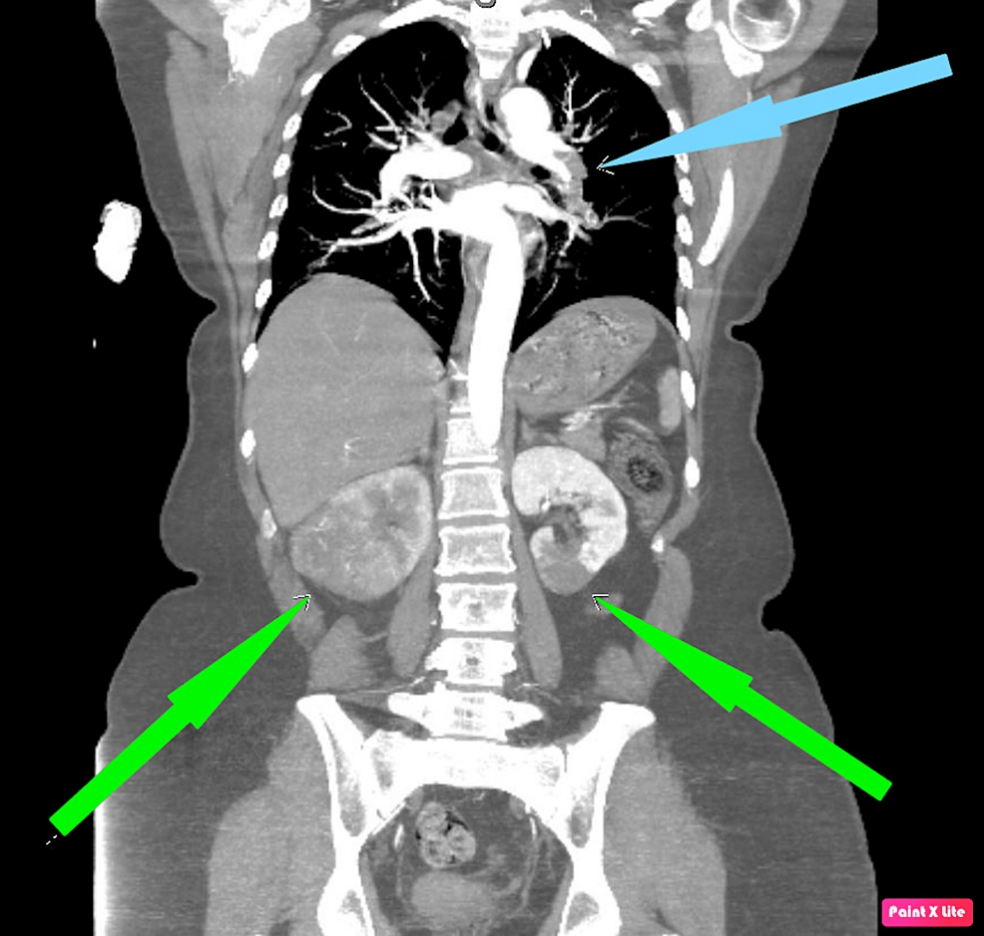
CT of the abdomen and pelvis showing left pulmonary embolus (blue arrow) and bilateral renal infarcts (green arrows) CT: computed tomography
